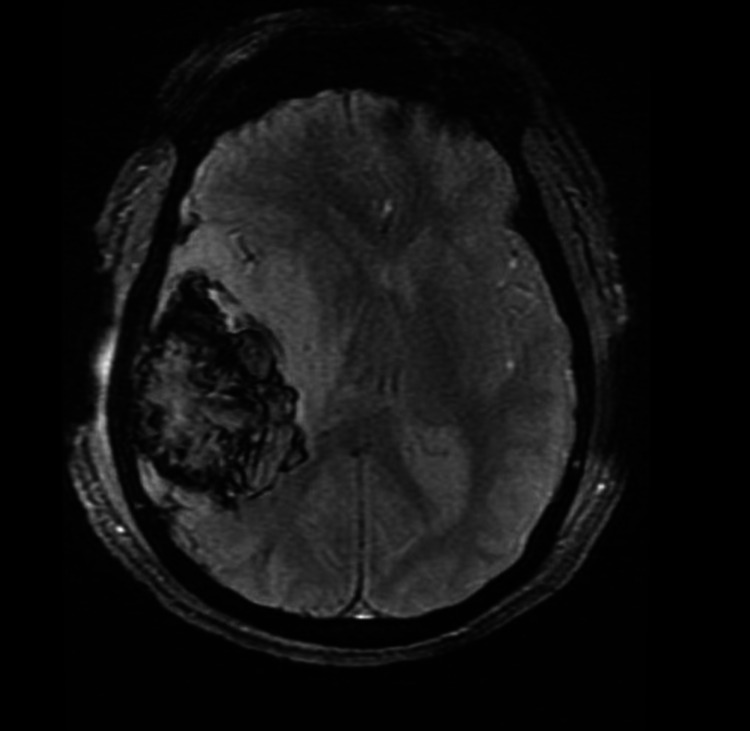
MRI of the brain suggestive of a right temporoparietal large hemorrhagic lesion
